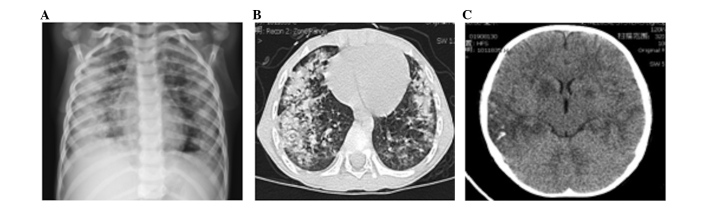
Chest X-ray and CT scan of the patient. (A) Chest X-ray revealing diffuse lung disease. (B) Lung CT scan showing diffuse parenchymal infiltration. (C) Head CT scan showing multiple low density areas in the left frontal lobe, right temporal lobe, parietal lobe, left parietal-occipital lobe, bilateral basal ganglia and trigone of the left lateral ventricle, with bleeding in certain areas. CT, computerized tomography.
